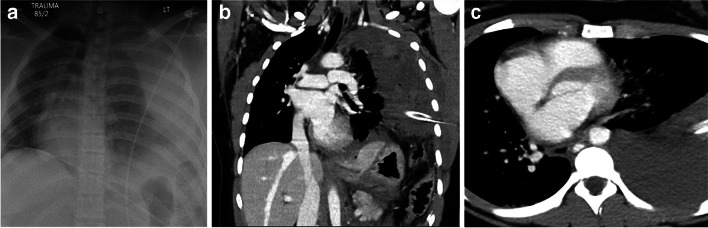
A 16-year-old boy with cardiac displacement due to diaphragmatic rupture. a Anteroposterior chest radiograph with electrodes over the thorax. (b) Coronal plane of a contrast-enhanced computed tomography (CT) with a chest tube in the left thorax and (c) CT in an axial plane. All images demonstrate displacement of the cardiomediastinal structures due to diaphragmatic rupture with herniation of the abdominal contents into the left hemithorax
